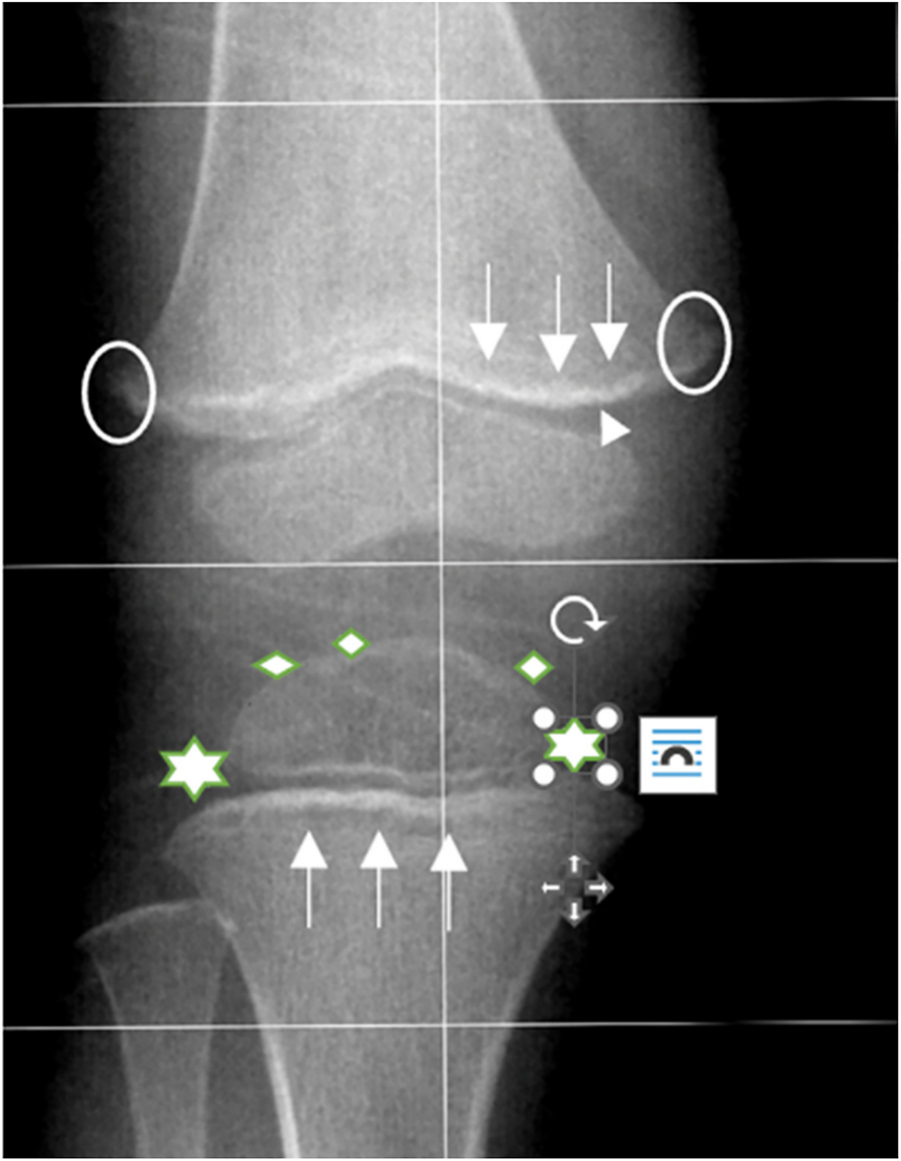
Scurvy in 3-year-old boy with skeletal pain (ID-08). Antero-posterior right knee radiography shows the classic radiographic signs described in literature: Dense zone of provisional calcification at edge of metaphysis (white line of Frankel) (head arrows), transverse radiolucent metaphyseal line (Trümmerfeld zone) (white arrows), circular calcification surrounding the osteoporotic epiphyseal center of ossification (Wimberger ring sign), and beaklike metaphyseal excrescences (Pelkan spurs, corner or angle sign) (white circles) [5].
